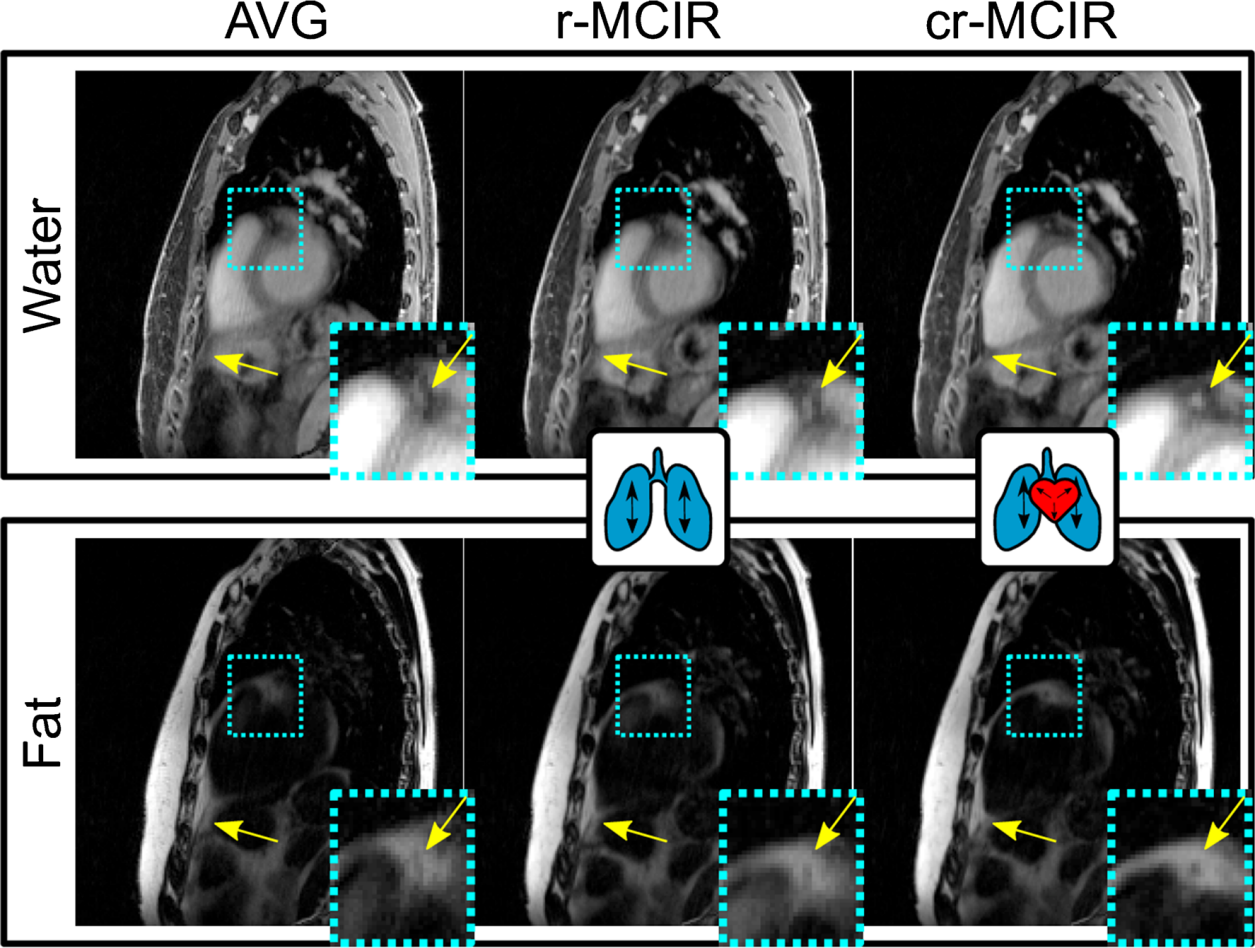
MR images in sagittal view of one exemplary dataset with reconstructed water (top) and fat (bottom) content. The motion-averaged reconstruction (AVG, left) is compared to respiratory (r-MCIR, centre) and cardiorespiratory (cr-MCIR, right) reconstruction. Cyan boxes indicate enlarged areas depicted in the lower right corner reconstruction. Image quality increases with the inclusion of more motion information from AVG over r-MCIR to cr-MCIR for both the water and fat images especially in the coronary arteries and apex (yellow arrows)
